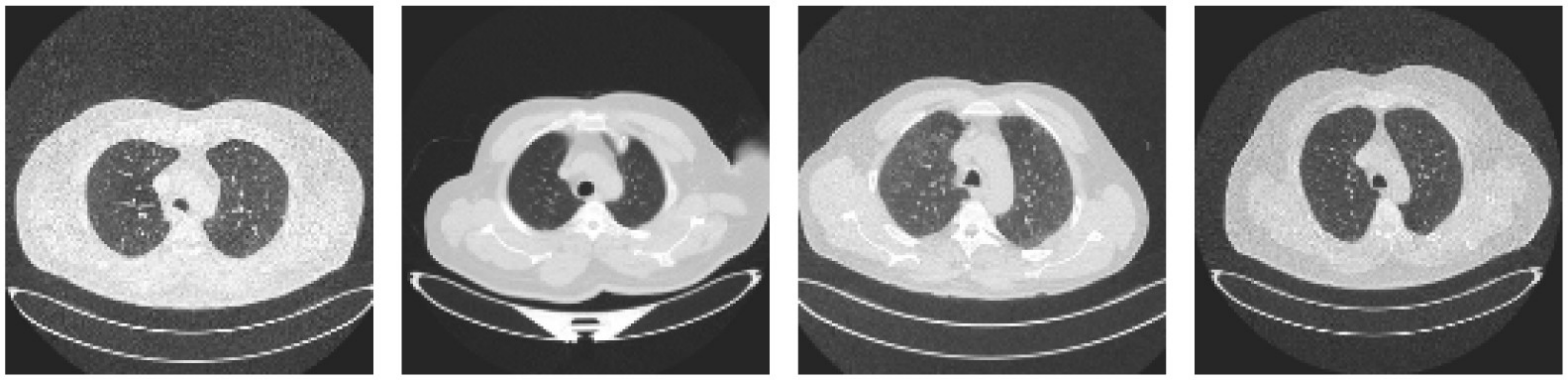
CT scan images showing noise and distortion, low-density contrast, and quality variation, respectively.
